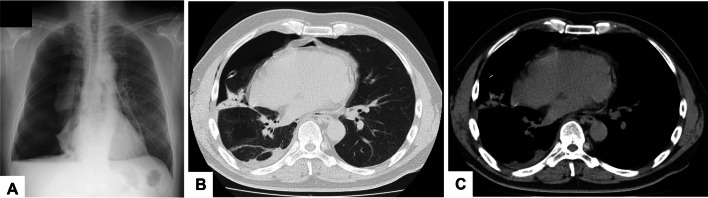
Chest radiography (A) shows a right lung collapse and a pneumopericardium on the left side. Computed tomography (CT) before surgery with lung window settings (B) and with mediastinal window settings (C) shows air in the pericardial sac and the right atrium was prolapsed into the right thoracic cavity
